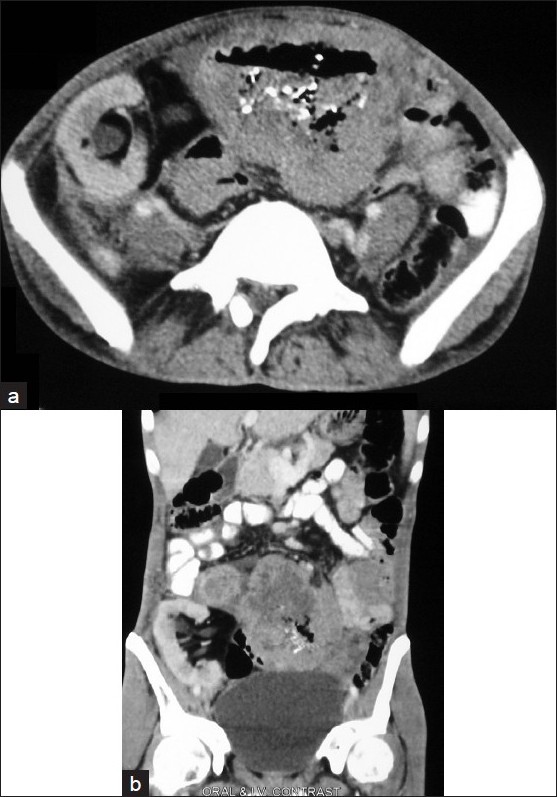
Contrast-enhanced axial (a) and coronal (b) computed tomography images in a 32-year-old male with abdominal lump
